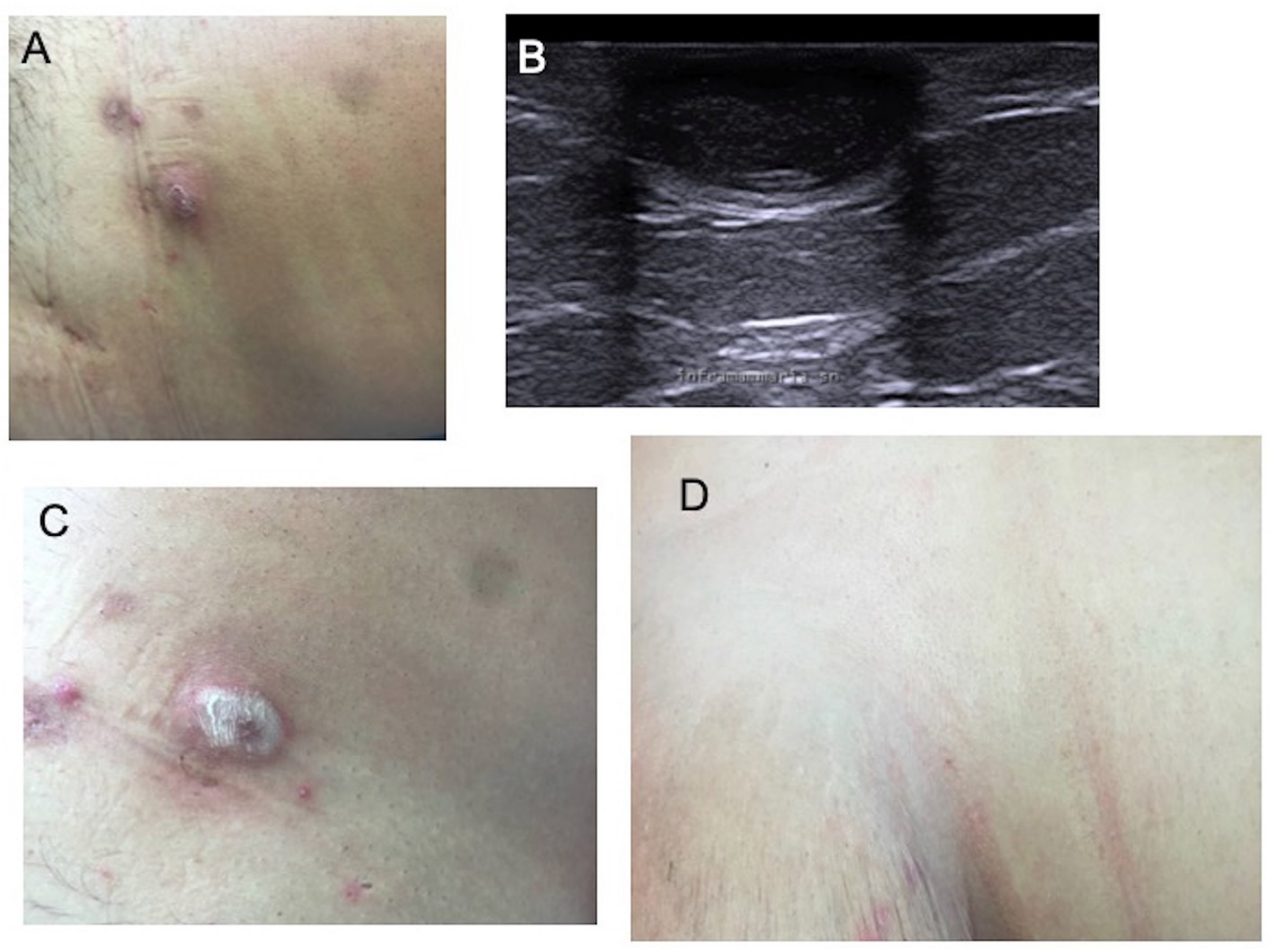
Nodular lesion of hidradenitis suppurativa of the right buttocks (A); ultrasound imaging documenting a roundish hypoechoic nodule in the soft tissue (B); immediately after cryotherapy (C); complete resolution of the lesion at 3-month follow-up (D).
